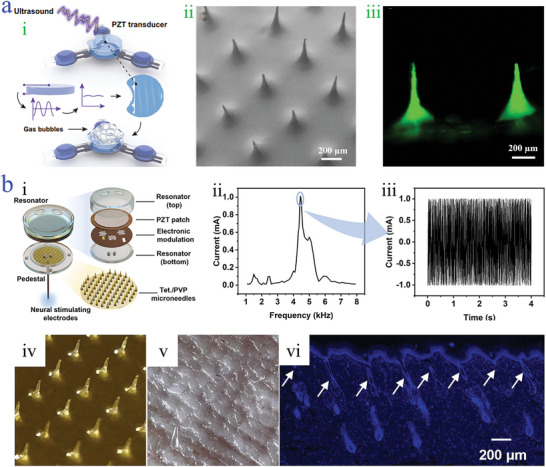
Piezoelectric‐driven microneedles. a) Scheme of the electrolysis reaction generated from ultrasonic of the riboflavin loaded PVP microneedle patch i); SEM image of the riboflavin loaded PVP microneedle array ii); fluorescence image of the PVP microneedle patch with drug loading iii).^[^
